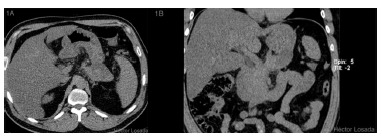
A) Axial unenhanced abdominal CT with collection contacting the posterior gastric Wall; B) coronal unenhanced abdominal CT with collection contacting the posterior gastric wall.
